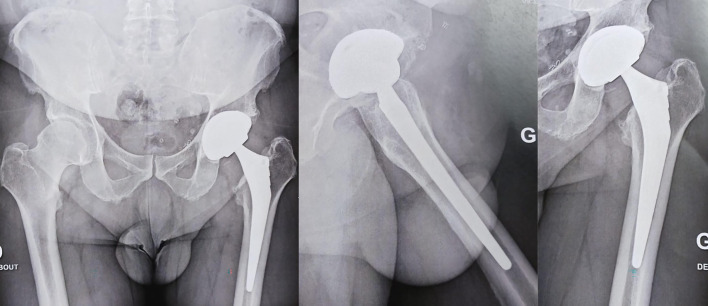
Postoperative X-ray at eight-year follow-up demonstrating implant stability.
